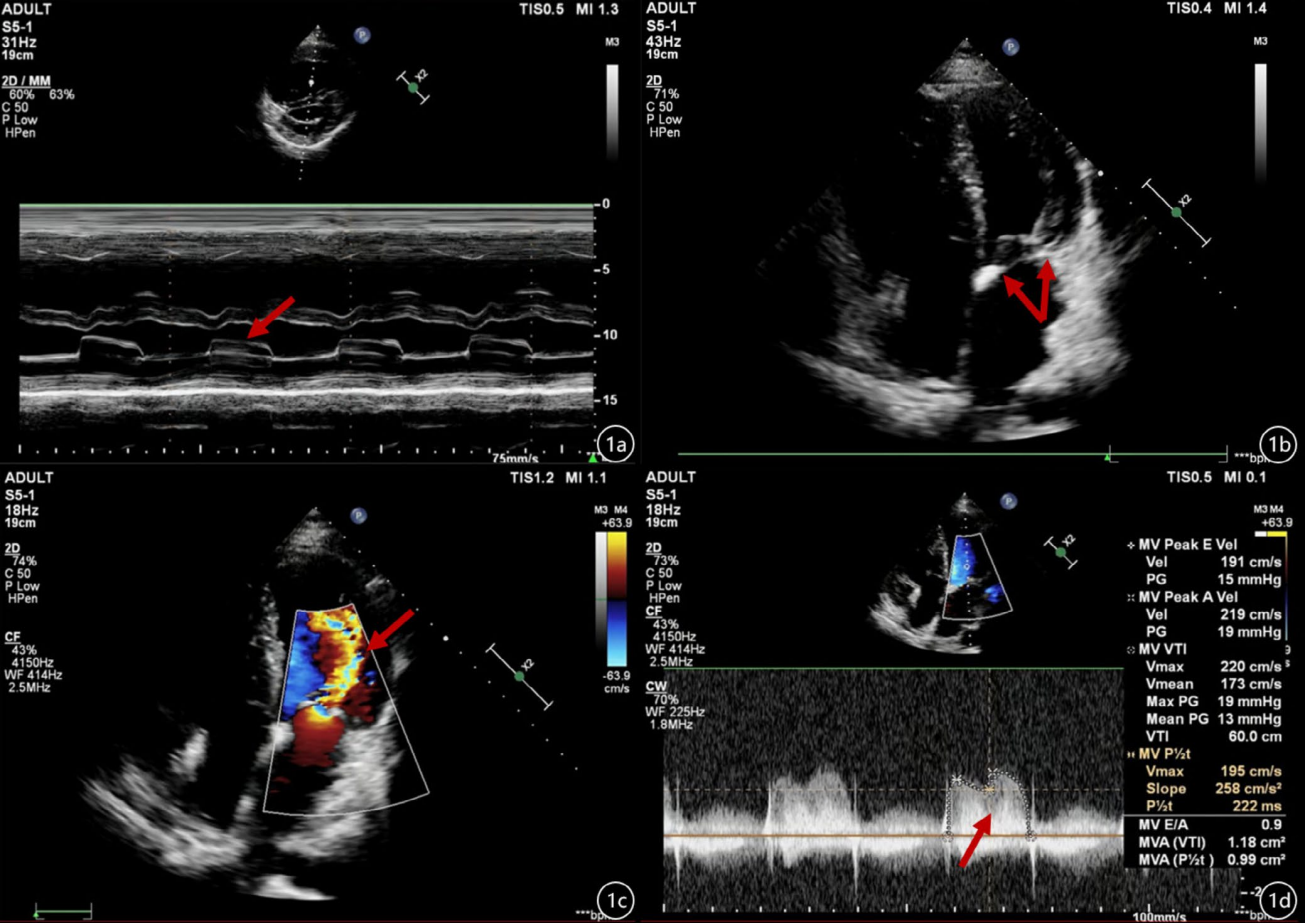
(a) Left ventricular long‐axis view, M‐mode echocardiography showed the wall‐like movement of the mitral valves. (b) Apical four‐chamber view, indicating heart enlargement. The mitral valves were thickened and adhered, with limited movement of the mitral valves on enhanced ultrasound imaging. There was a partial absence of chordae tendineae in both anterior and posterior mitral valve leaflets, and the papillary muscles in the two groups were close together and partially fused. (c) Doppler Color, the effective valve orifice area was reduced, resulting in hemodynamic changes similar to parachute deformity; a multicolored shunt flow signal was seen on mitral regurgitation. (d) The effective orifice area of the stenotic mitral valve was 0.99 cm2 (P1/2t), which resulted in hemodynamic changes similar to those of parachute deformity with a peak diastolic velocity of 2.0 m/s and a peak across mitral valve pressure of 19 mmHg, and a mean gradient of 13 mmHg.
